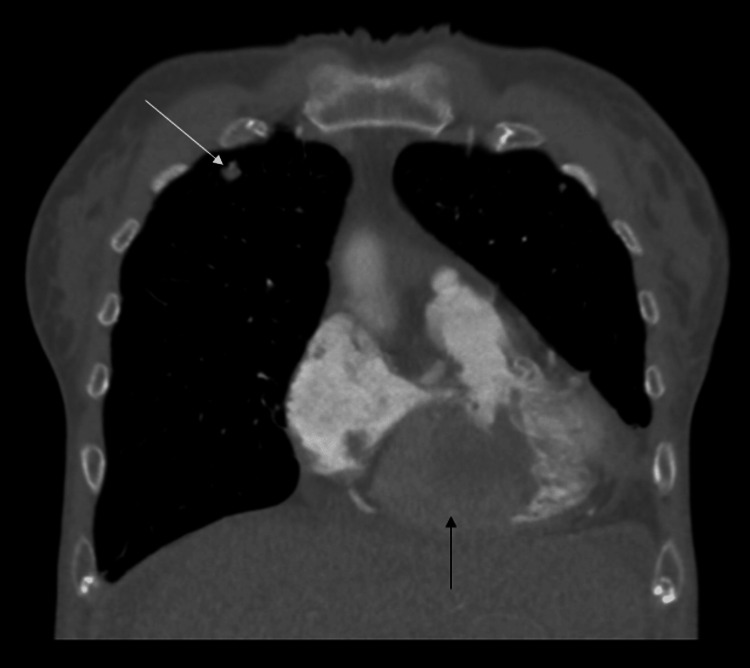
CT chest with contrast in the coronal plane displaying the right ventricular mass (black arrow) and right upper lobe nodule (white arrow).
